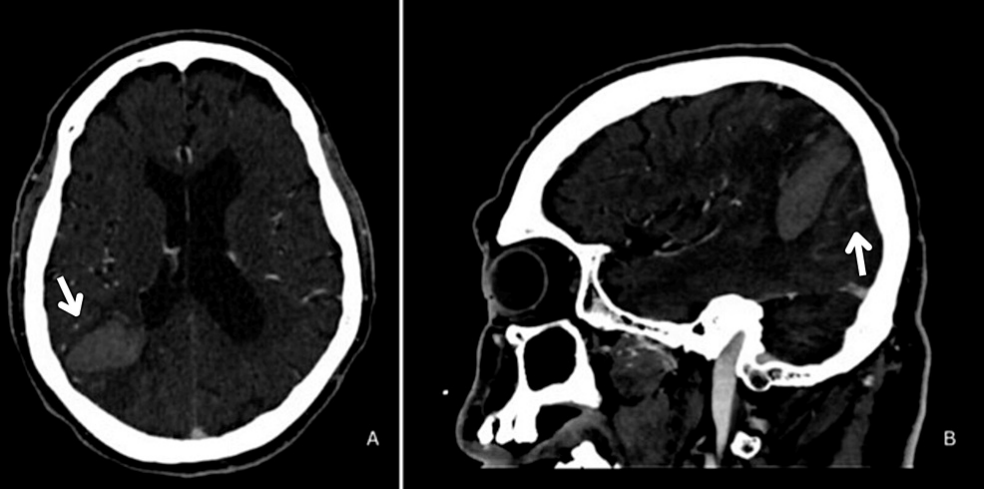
Computed tomography angiography with contrast Axial (A) and sagittal (B) computed tomography angiography (CTA) with venous contamination of the head and neck demonstrating displacement of the vascular structures surrounding the right parietal intraparenchymal hematoma due to mass effect (white arrows).
